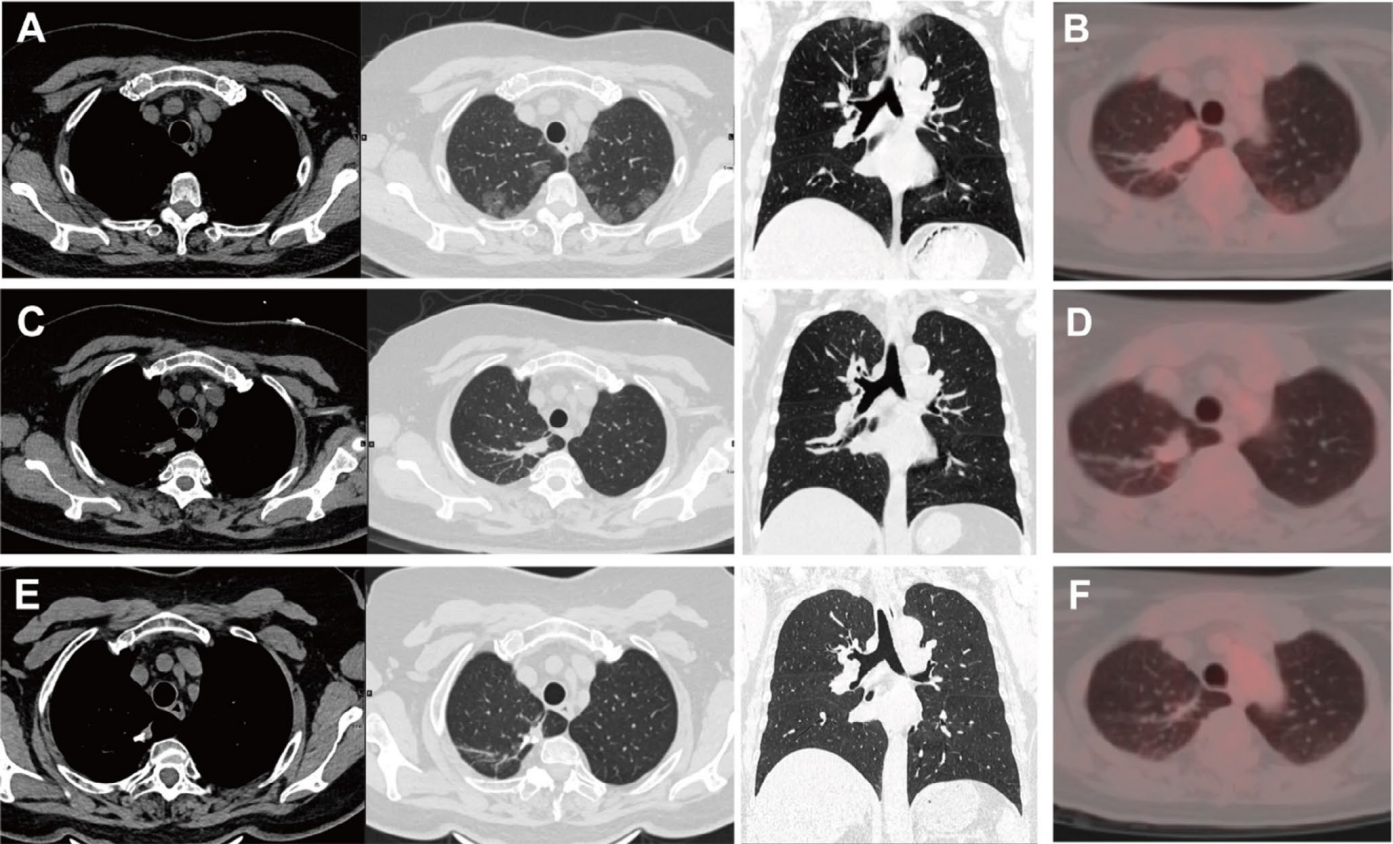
(A) Chest CT images before chemotherapy showing multiple GGOs beneath the pleura in the bilateral upper lobes of the lungs; (B) FDG PET/CT images before chemotherapy; (C, D) chest CT images and FDG PET/CT images after the first round of chemotherapy showing disappearance of lesions and no FDG uptake; (E, F) chest CT images and FDG PET/CT images after the second round of chemotherapy showing disappearance of the lesions and no FDG uptake.
